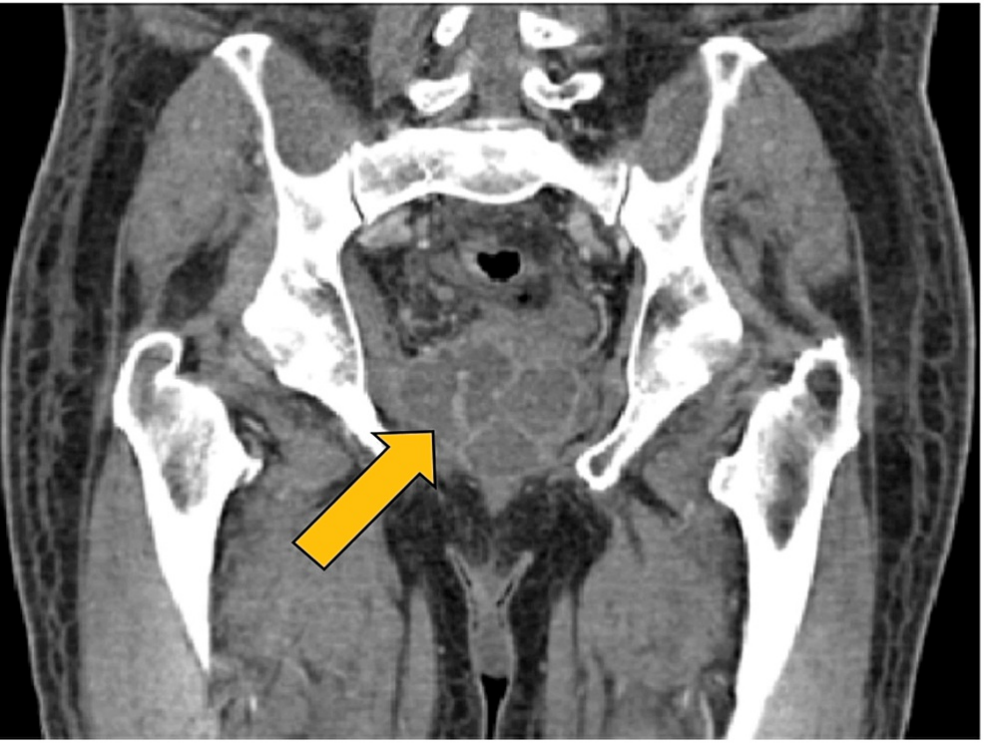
Coronal CECT showing multiloculated hypoechoic lesions with enhancing walls in the prostate CECT: Contrast-enhanced computed tomography.
